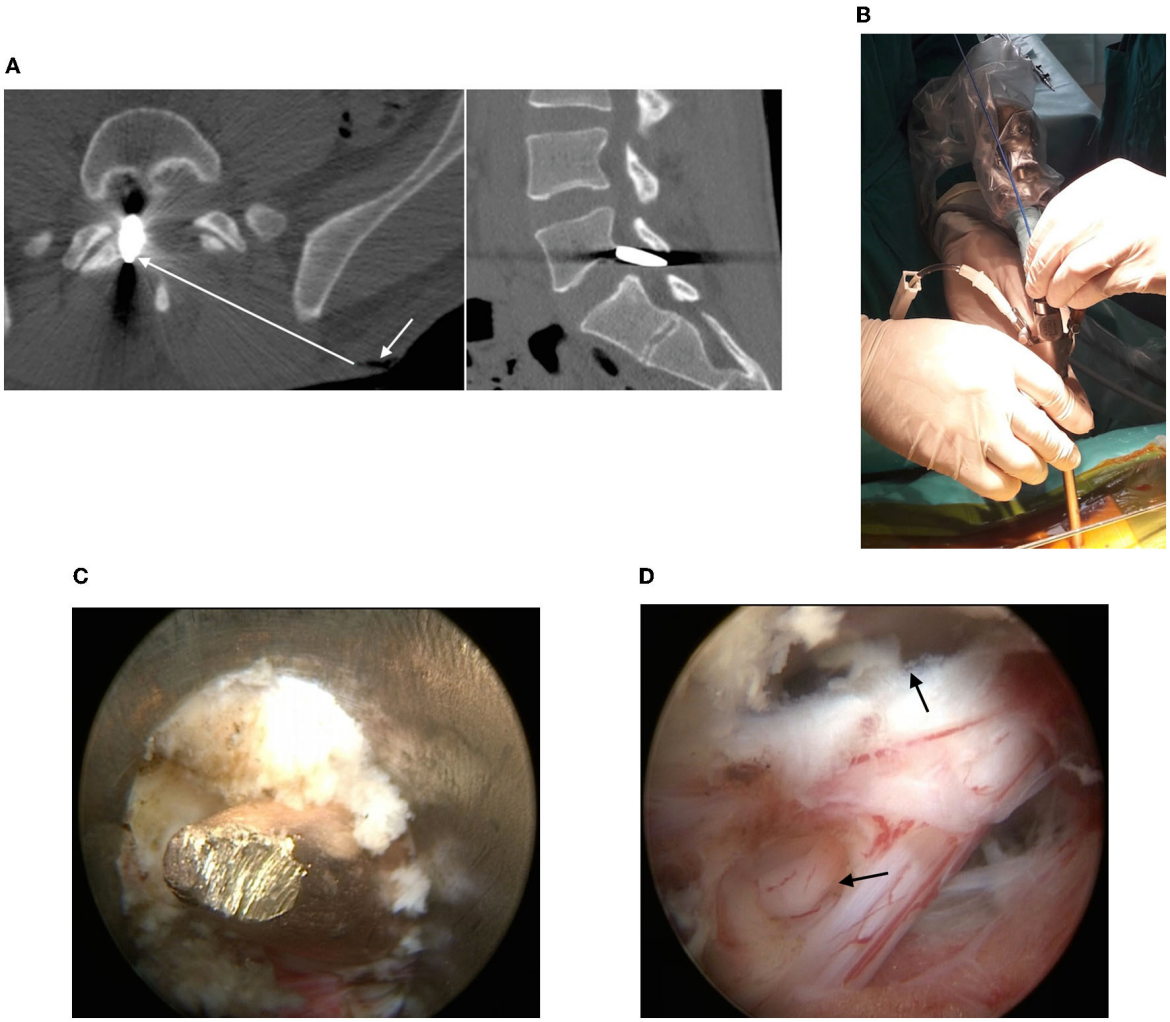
(A) CT scans of the lumbar spine: metallic foreign body (bullet) in the right half of the spinal canal (the arrow shows the inlet of the gunshot wound, the line shows an approximate trajectory of the bullet through the soft tissues); (B) View of the working port and endoscope; (C) Bullet mobilization in a yellow ligament defect; (D) Defects in the dura mater around the nerve root cuff and dural sac (see arrows).
